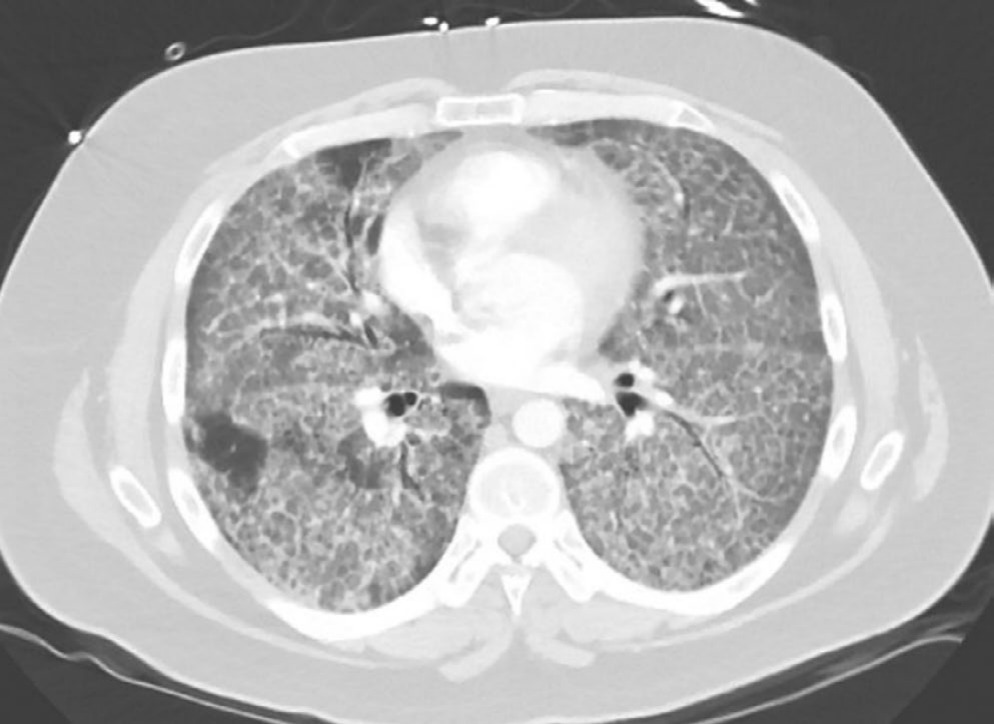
CT scan of the lungs showing widespread ground‐glass opacities and thickened septal lines in all five lobes, described as ‘crazy paving pattern’.
